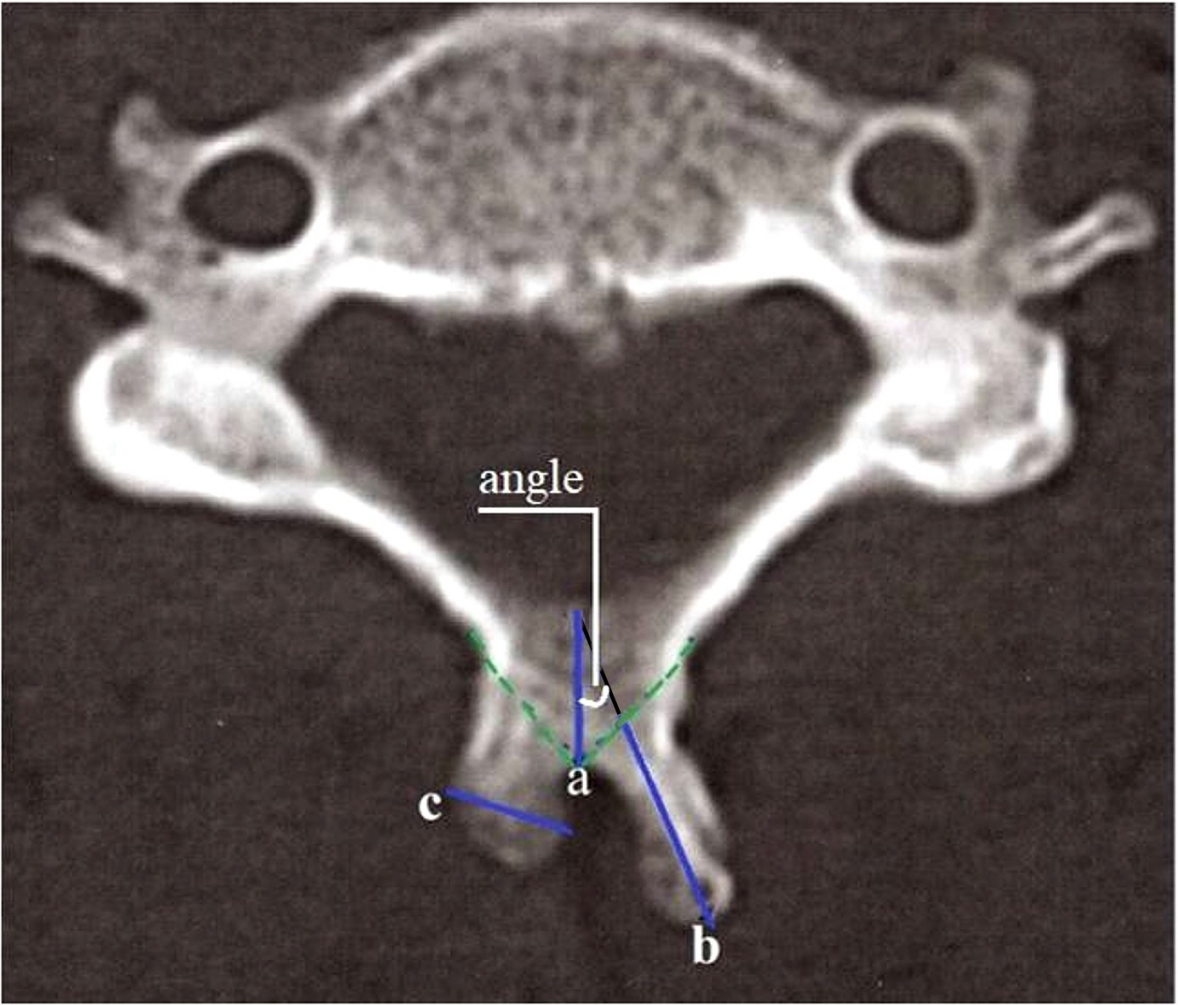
Defined and measured dimensions of the spinous processes–a zoomed view. a—length of the spinous process base; b—length of the spinous process branch; c—width of the spinous process branch; “angle”–branching angle. Multidetector Computed Tomography (MDCT).
